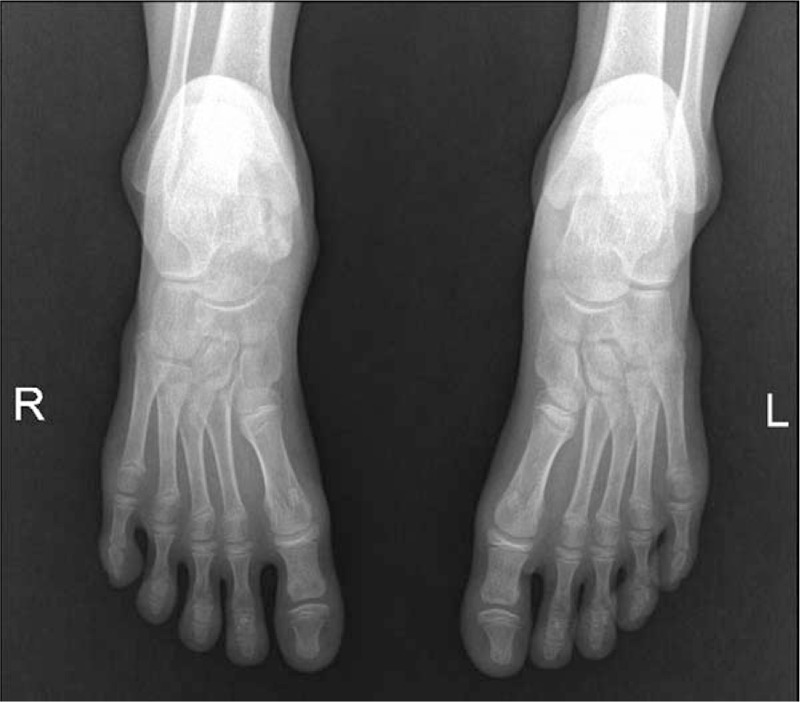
Anteroposterior X-ray view and lateral film of the feet showed a bone mass in the medial talus, with a smooth edge. The accessory scaphoid bone can be observed in the left foot.
